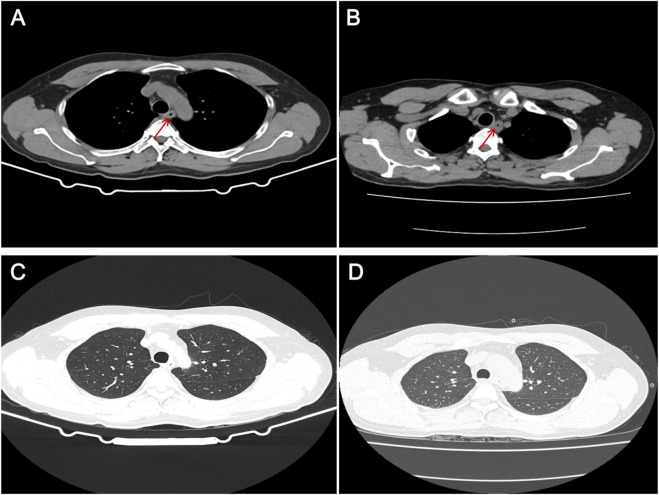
Serial thoracic computed tomography (CT) scans before and after rituximab rechallenge. (A) Mediastinal window (15 August 2025) shows normal esophageal wall thickness (red arrow). (B) Mediastinal window (22 August 2025) demonstrates focal esophageal wall thickening and edema with minimal peri-esophageal stranding (red arrow), consistent with angioedema. (C) Lung window (15 August 2025) shows clear lung fields without consolidation or ground-glass opacities. (D) Lung window (22 August 2025) remains clear, with no radiographic evidence of pulmonary infection or infiltrates. Comparison confirms the interval development of esophageal wall edema without new pulmonary findings.
